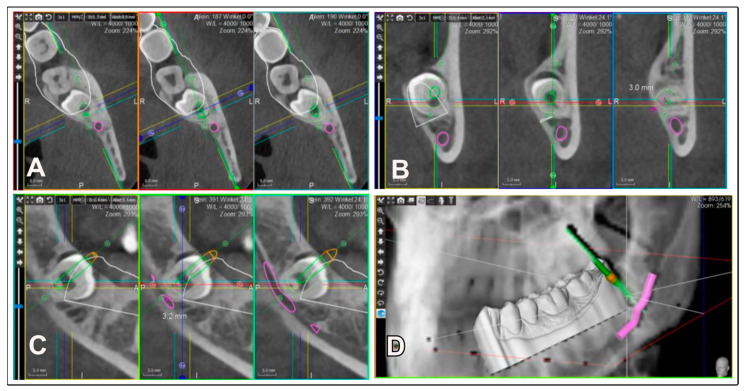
Three-dimensional planning of the surgical access for the IFT in CBCT. The target zone, i.e., the furcation area, is planned in three spatial planes: axial, coronal, and sagittal. (A): axial view, (B): coronal view, (C): sagittal view, (D): 3D representation of the direction and depth of the access to the furcation area.
